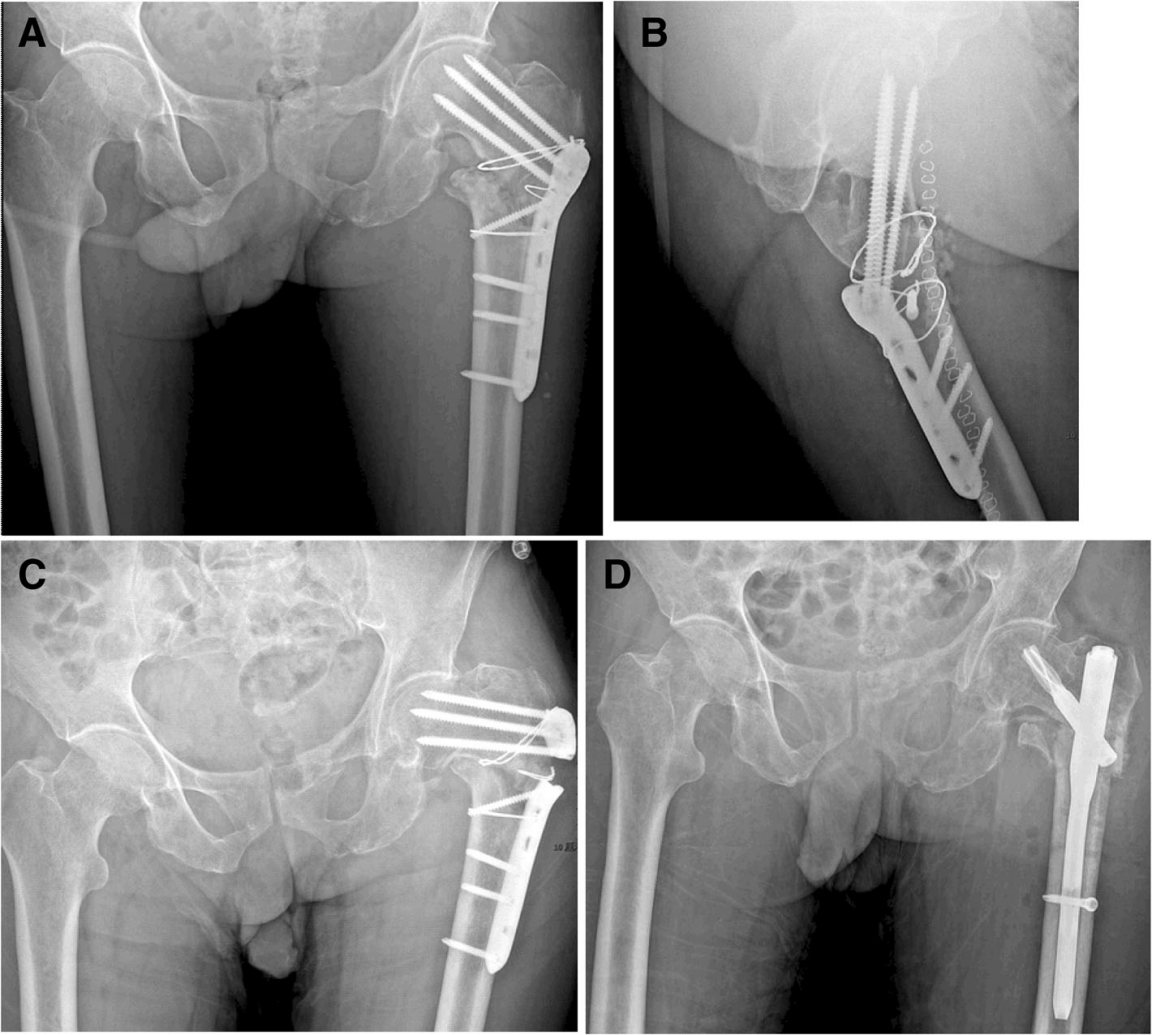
A 78-year-old male with AO/OTA type 31A3.3 experienced plate breakage. a, b Postoperative anterio-posterior and lateral radiographs. c Plate breakage and varus collapse 10 weeks after surgery. d Postoperative radiograph of revision surgery of PFNA
